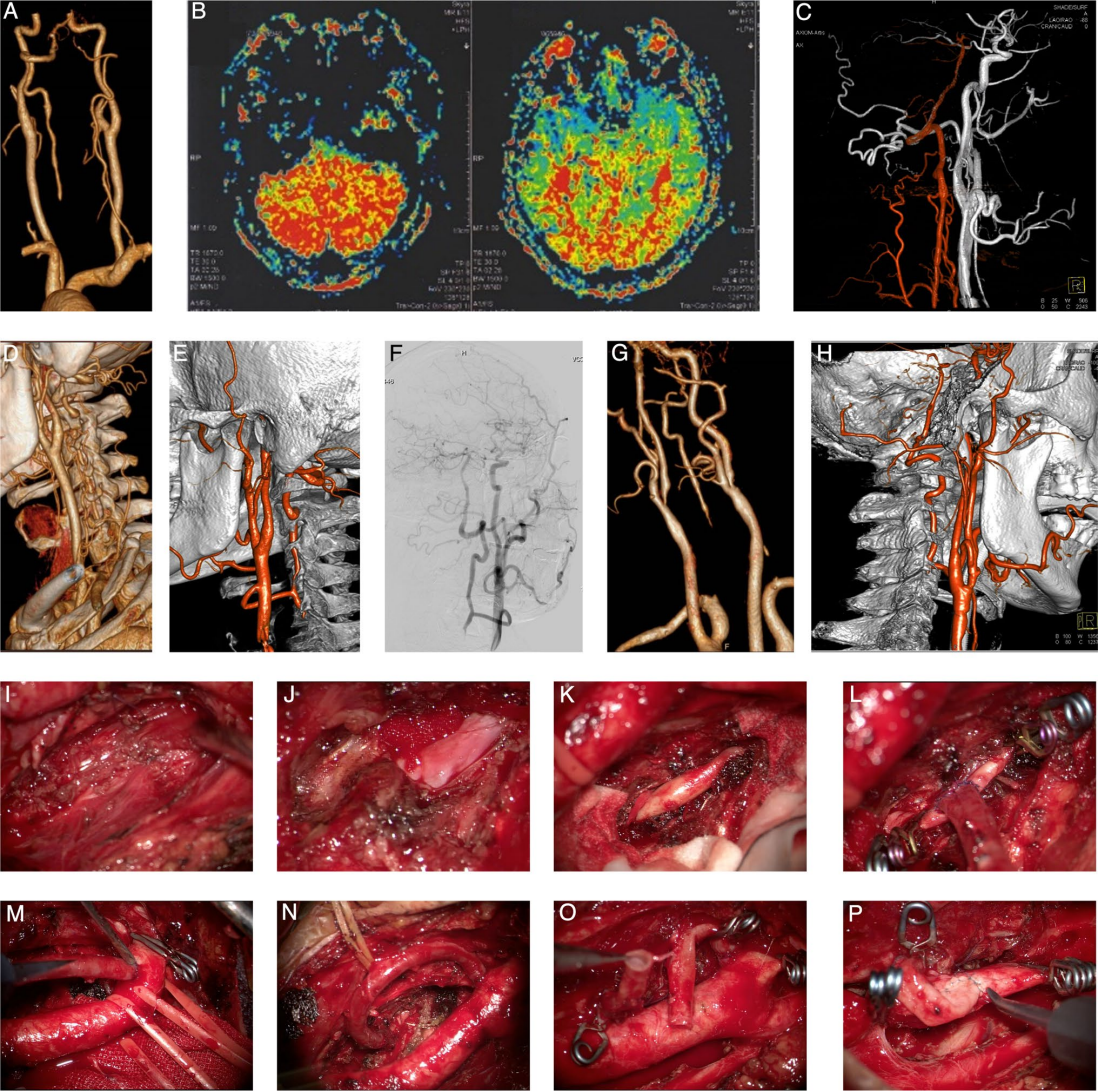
Representative cases of bilateral VA occlusions (Type I). A–F (Case 6): A: CTA reveals bilateral VA occlusions (left extracranial, right intracranial). B: MR perfusion indicates significant posterior circulation hypoperfusion. C: Preoperative angiography shows distal VA filling via OA and AA/DA collateral pathways. D: CTA demonstrates surgical anatomy of the cervical region. E: After initial occlusion, the donor site was switched from ECA to CCA, with subsequent angiography confirming patency. F: Postoperative angiography shows enhanced and timely filling of the distal vasculature. G–H (Case 7): G: CTA displays bilateral VA occlusions and severe ipsilateral ICA origin stenosis. H: Combined CEA and V2 bypass were performed utilizing overlapping surgical exposures. I–P (Intraoperative Sequence): I: The prevertebral aponeurosis is opened and retracted anteriorly. J: The intertransverse segment of V2 is identified deep to the muscle layer. K: The VA is exposed after unroofing the transverse foramen; hemostasis is aided with gelatin sponge or Surgicel. L: End-to-side anastomosis between the radial graft and V2 is completed. M: The graft is transposed to the pre-carotid space and anastomosed to the ECA. N: Final view after bypass completion. O: The occluded graft is examined and flushed after unsuccessful intraoperative angiography. P: Graft reimplantation to the CCA is performed as a salvage procedure
